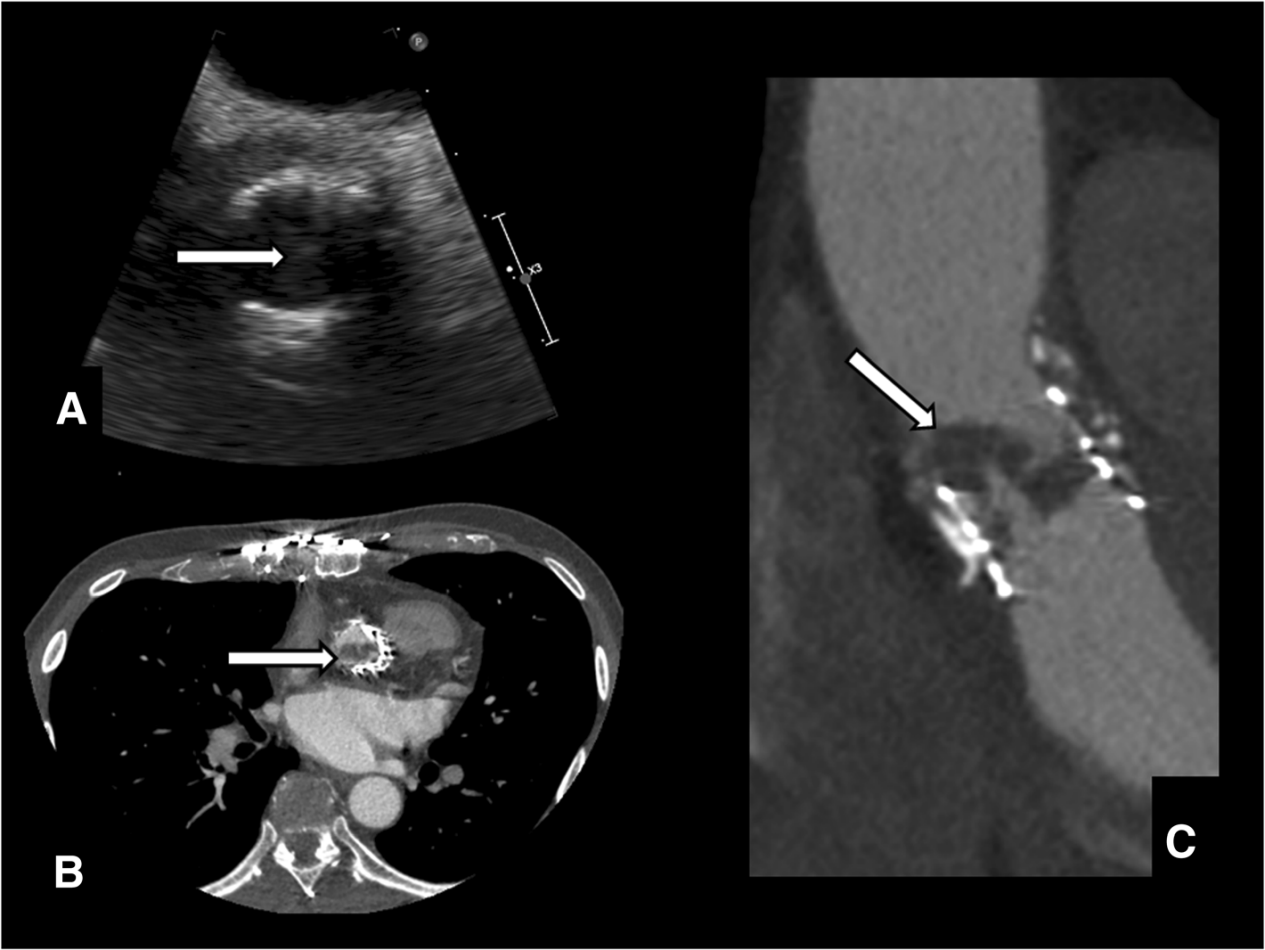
a The echocardiogram was suggestive for valve thrombosis (*arrow*). b & c. The finding was confirmed on thoracic computed tomography (*arrows*)
